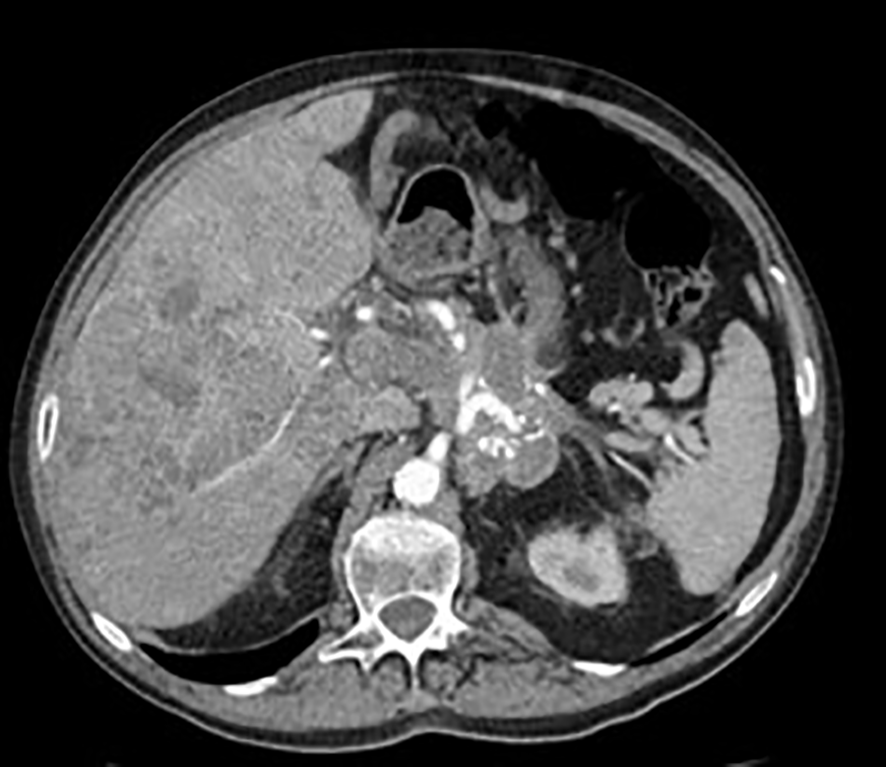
Abdominal CT scan performed at the time of insulinoma diagnosis showing extensive liver metastases.
